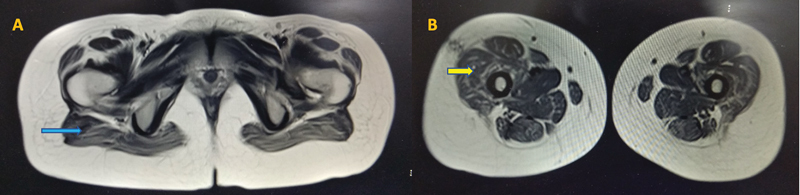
Muscle MRI of the patient: (A) and (B) show muscle MRI–T2 sequences that show fatty infiltration with atrophy of the glutei (blue arrow) and quadriceps (yellow arrow). MRI, magnetic resonance imaging.
